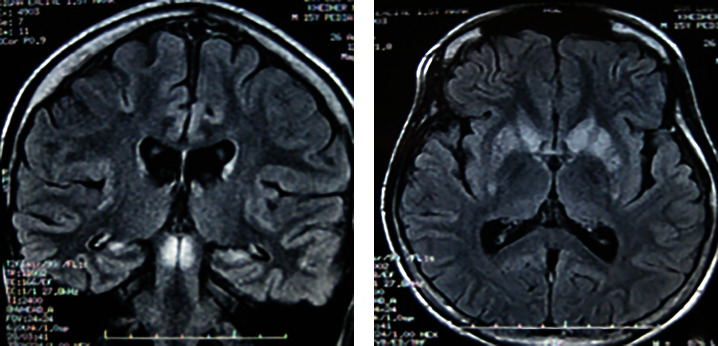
Cranial MRI revealed bilateral and symmetrical hyperintense lesions of the mesencephalon and lenticular and caudate nucleus compatible with LS (transverse view).
